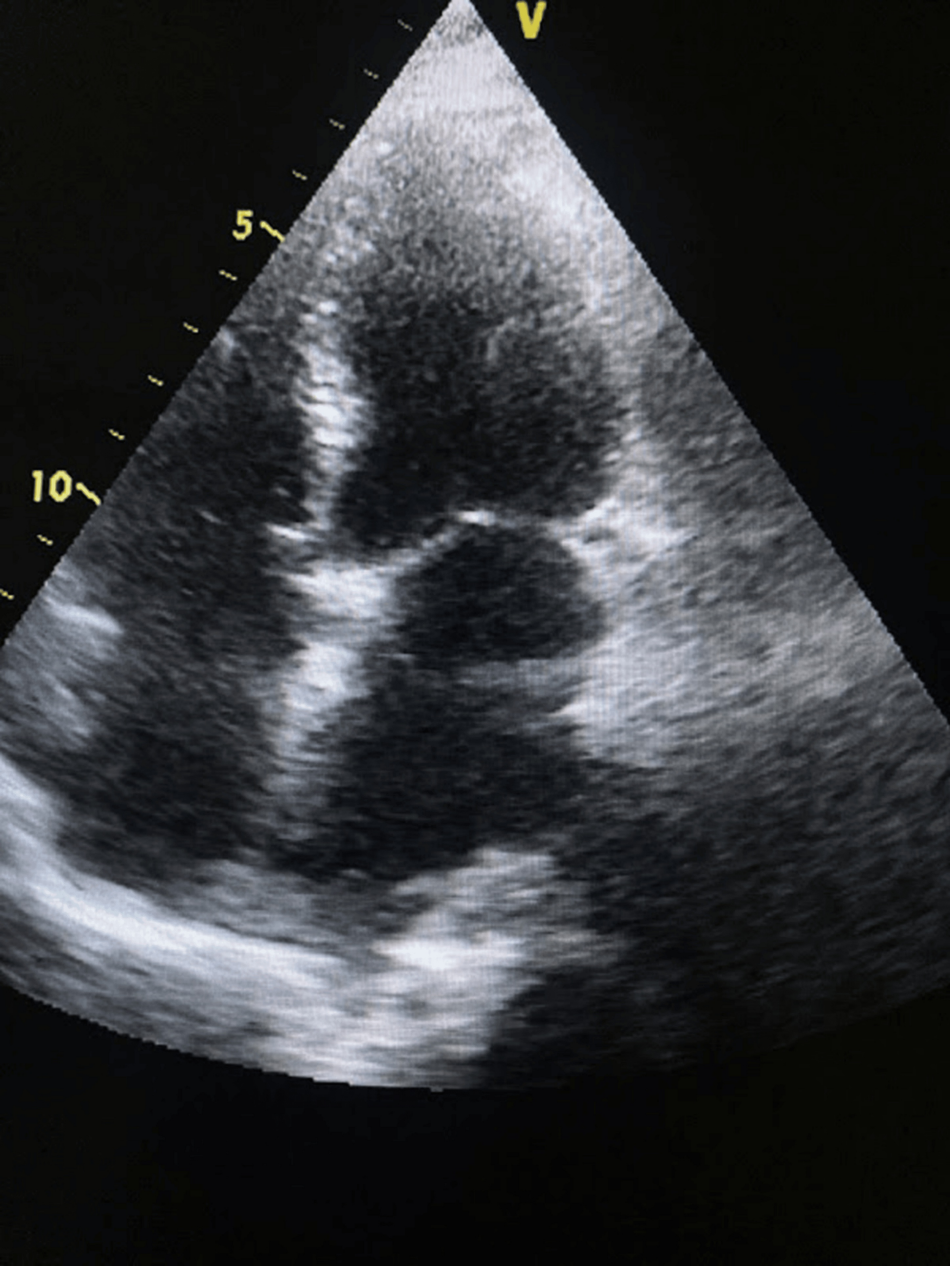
Transthoracic echocardiography (performed on the fourth postoperative day) (apical four-chamber view) showing no residual myxoma tissue
